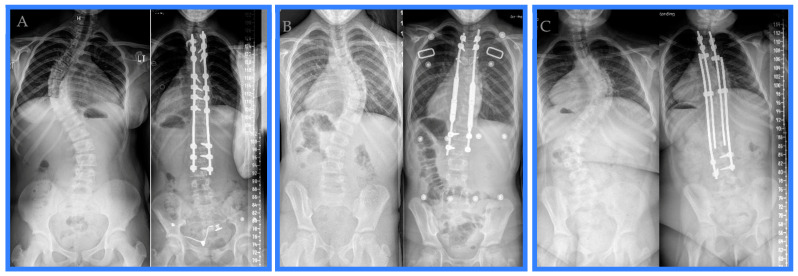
Pre-operative and first post-operative appointment radiographs exemplifying a (A) posterior spinal fusion, (B) magnetically controlled growing rod, and (C) traditional growing rod treatment of juvenile idiopathic scoliosis during the study period.
